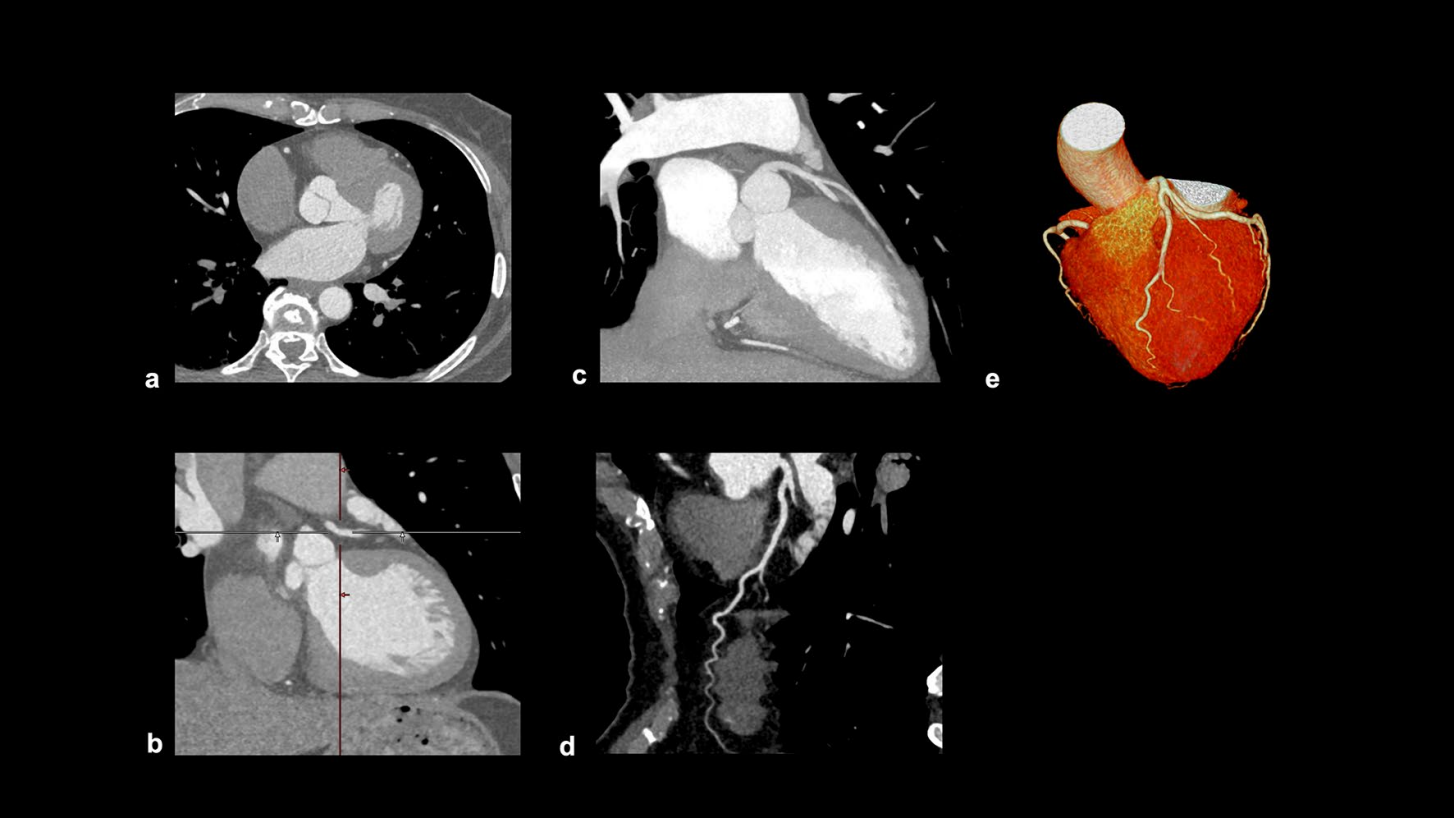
Recommended CCTA Images post-processing format. Recommended CCTA images post-processing format are as follows; a axial images, b multiplanar reformation (MPR), c maximum intensity projection (MIP), d curved planar reformation (CPR), and e volume rendering reformation (VR). *CCTA* coronary computed tomography angiography
